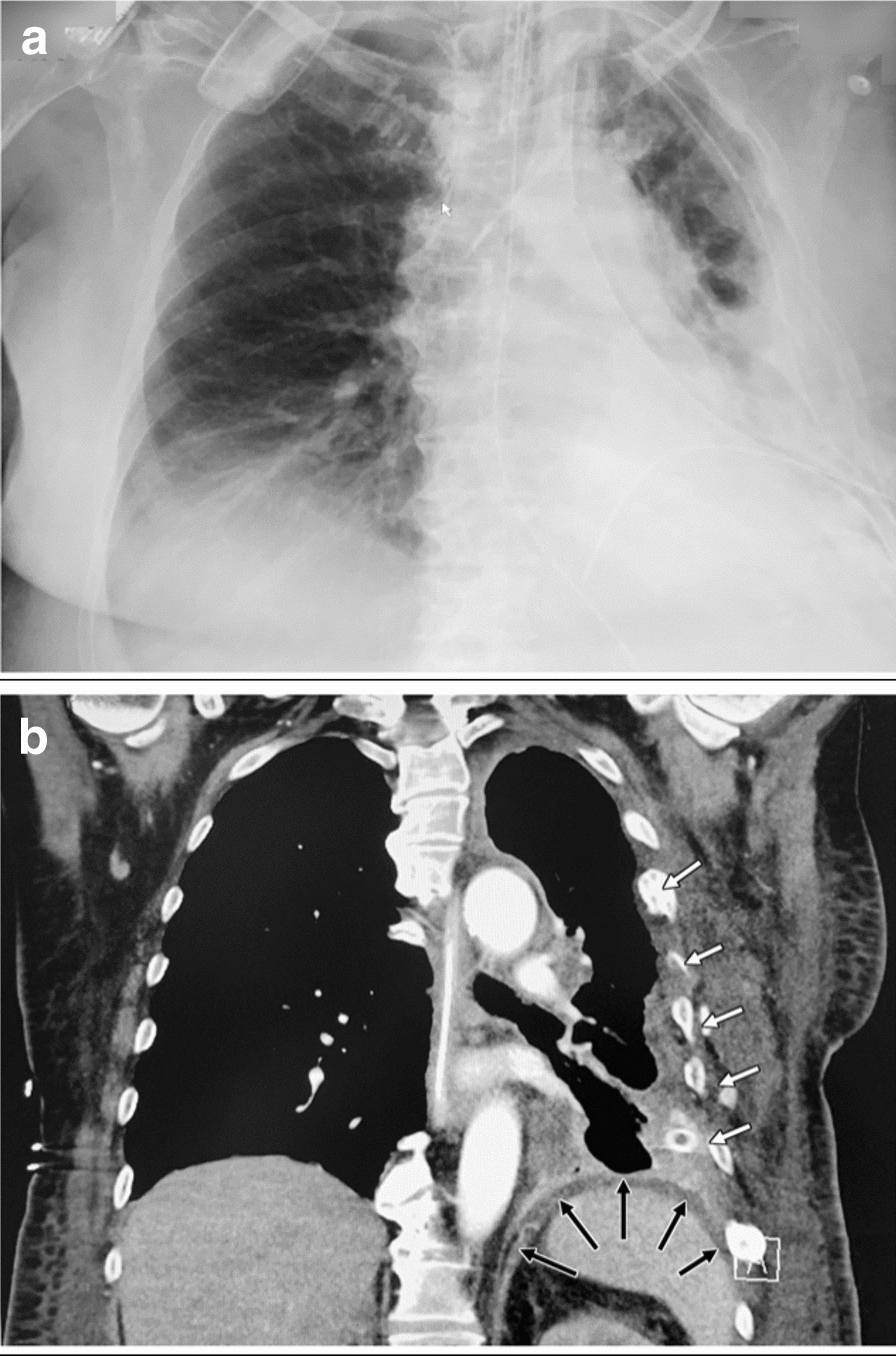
Radiological imaging on day 20. Chest X-ray examination (a) and thoraco-abdominal CT scan (b): reaccumulation of the left pleural empyema. The diaphragm stayed intact (black arrows) over the whole clinical course subsequent to reconstruction during initial surgery on day 1. White arrows indicate remnants of the healed rib fractures as a consequence of the bicycle trauma 7 months prior to admission for feco-pneumothorax
